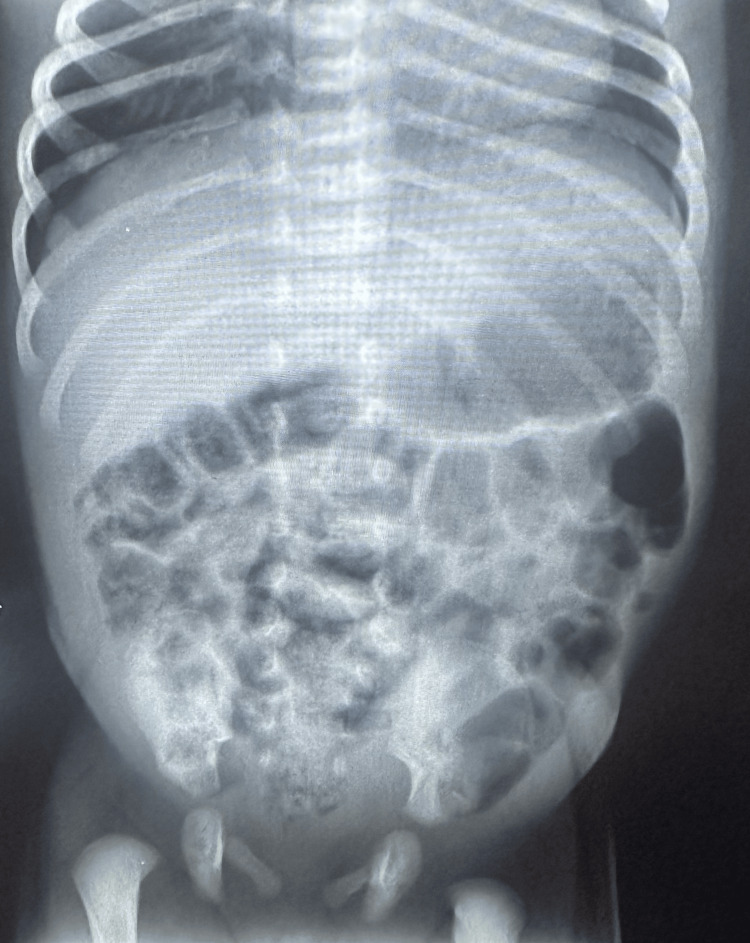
Neonatal abdominal radiograph Abdominal radiograph of a seven-day-old neonate without acute findings of obstruction, pneumatosis, or free intraperitoneal air.
